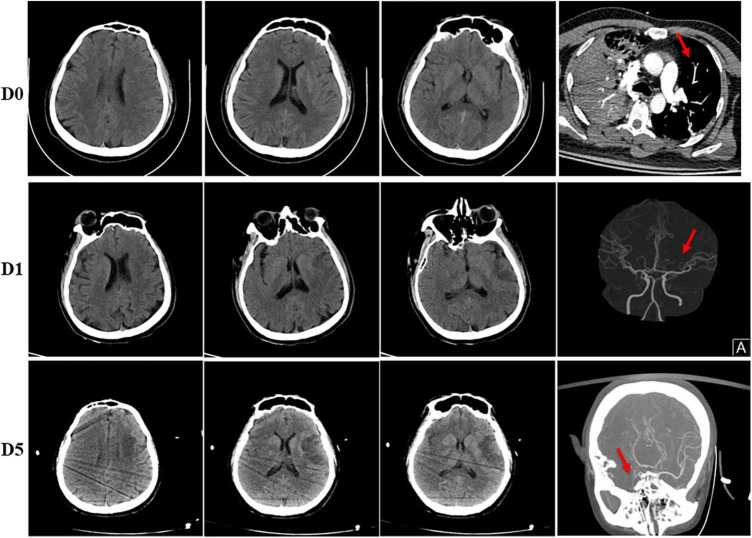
Head imaging of the patient. (D0) Normal head CT before admitted. His CTPA showed embolism of left pulmonary artery branches (red arrow). (D1) Ischemic infarction of left frontal and temporal lobes was shown in his head CT on day 1 after admitted, CTA examination showed stenosis of the left MCA (red arrow). (D5) CT scan of head on day 5 showed no obvious difference compared to day 1, however, CTA examination showed occlusion of right MCA (red arrow).
